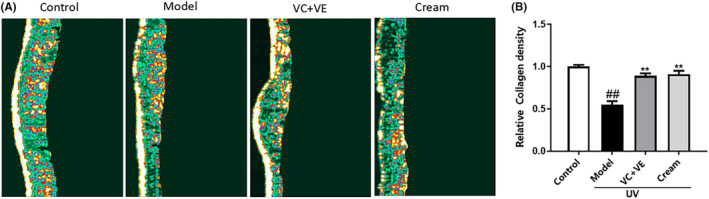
Effect of cream treatment on UV‐induced collagen density in ex‐vivo human skin was evaluated by skin ultrasound. (A) Compared to the control group, the model group exhibited a reduction in highlighted areas, indicating a decrease in collagen density. In contrast, both the sample cream and positive control resulted in an increase in highlighted areas compared to the model group, suggesting enhanced collagen density. (B) The relative collagen density in each group. Values are expressed as means ± SD (*n* = 3). ^###^
